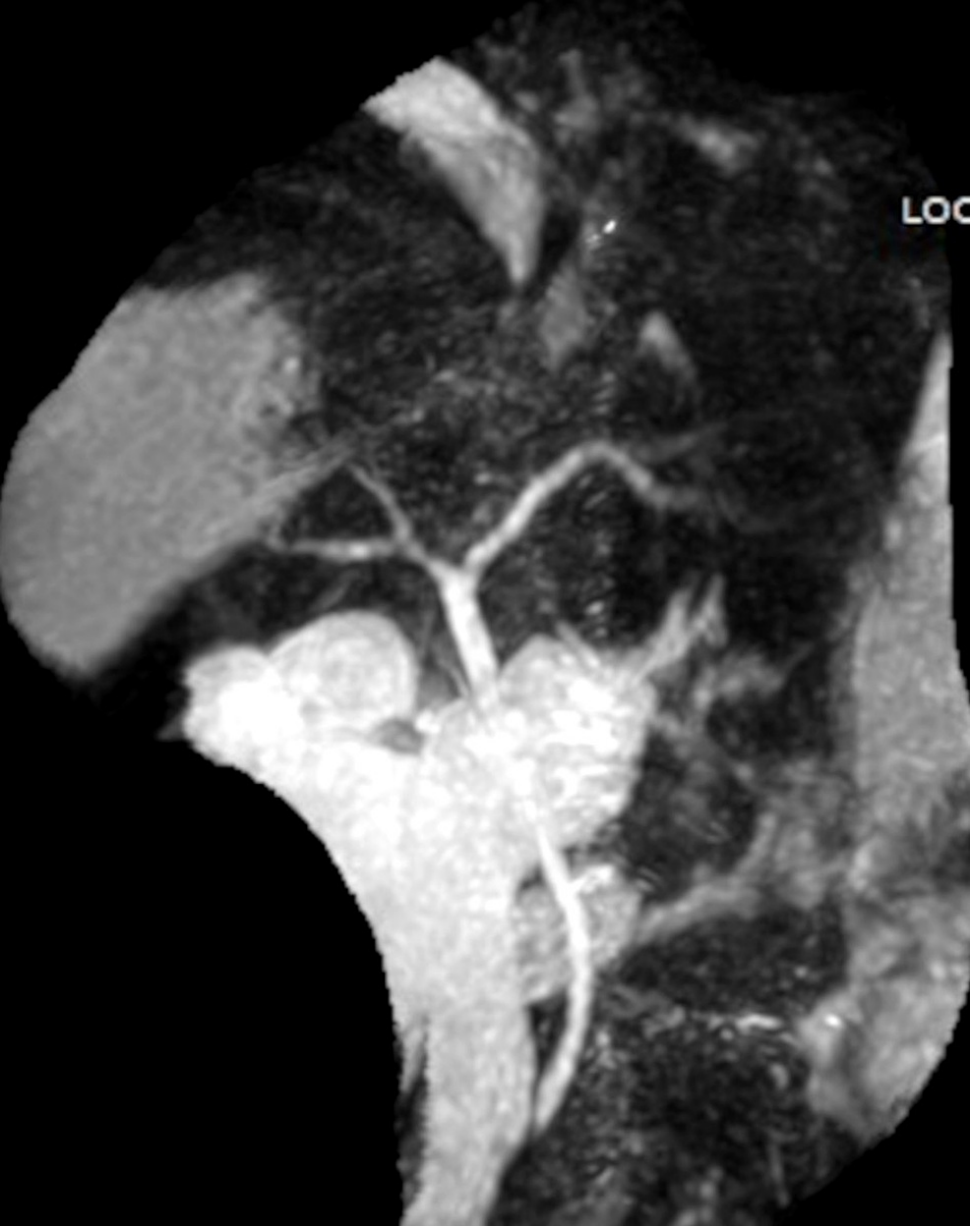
Magnetic resonance image of the biliary tree showing an intact intra- and extrahepatic biliary tree without stones or strictures.
